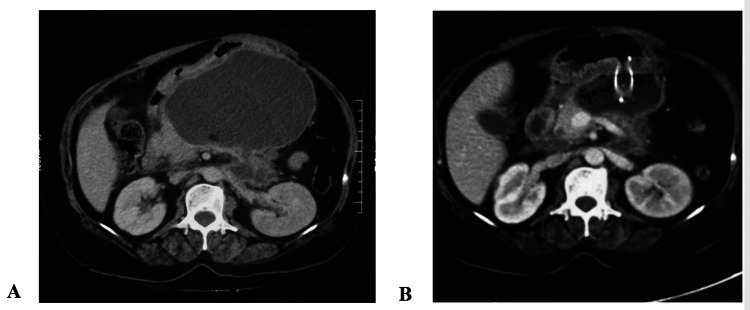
A: Contrast-enhanced computed tomography showing large infected walled-off necrosis in lesser sac compressing stomach; B: Contrast-enhanced computed tomography of the same patient after 72 hours of drainage showing significant reduction in size of walled-off necrosis after endoscopic drainage with metal stent
